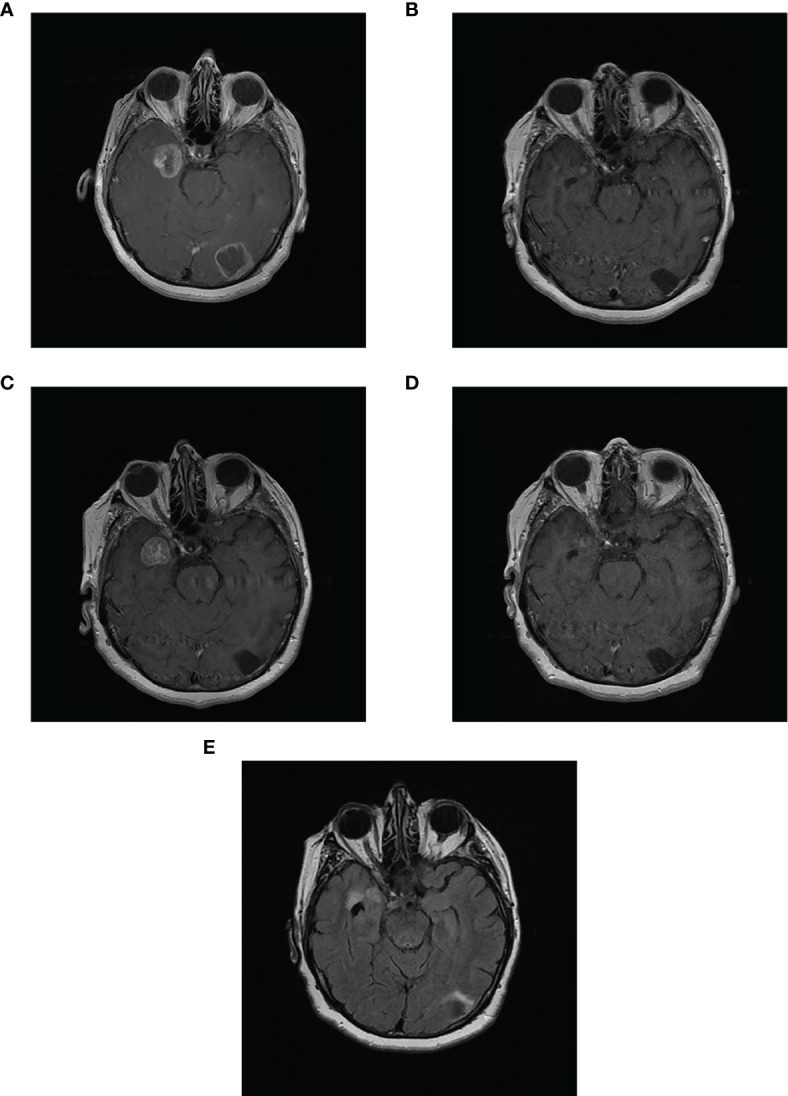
Complete response of 2 asymptomatic brain metastases in patient case 3. Magnetic resonance imaging scans of patient case 3: (A) with 2 brain metastases in the left occipital lobe and right temporal lobe prior to starting avelumab (May 9, 2018); (B) Complete response in occipital lobe and partial response in temporal lobe metastases after 8 months of avelumab (January 21, 2019); (C) PD in temporal lobe metastasis after 10 months of avelumab (April 29, 2019; patient began concurrent SRS on May 31, 2019); (D) substantial decrease in size of temporal lobe metastasis after SRS and 15 months of avelumab (September 4, 2019); (E) Complete response in both metastases (June 16, 2020; avelumab treatment stopped on February 24, 2020).
